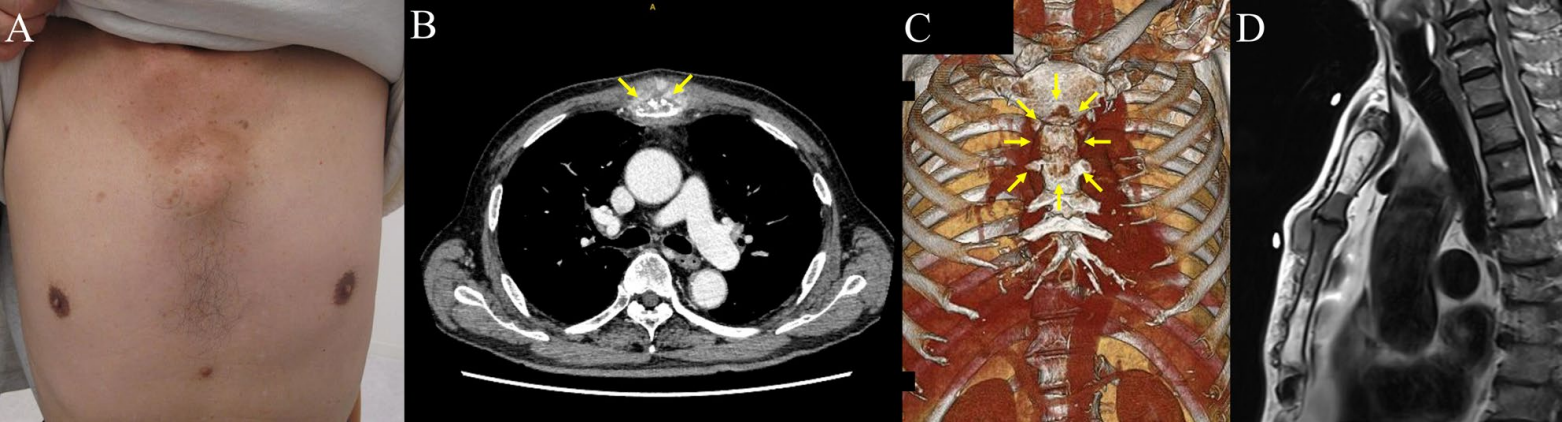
Preoperative gross findings and imaging of the sternal tumor. (A) Gross appearance. (B) CT image showing a 3-cm tumor in the sternum with osteolytic features. Arrows indicate the osteolytic areas. (C) Three-dimensional reconstructed CT image of the sternal tumor. Arrows indicate the tumor. (D) Magnetic resonance imaging revealing tumor invasion into both the manubrium and the body of the sternum. Abbreviations: CT, computed tomography.
